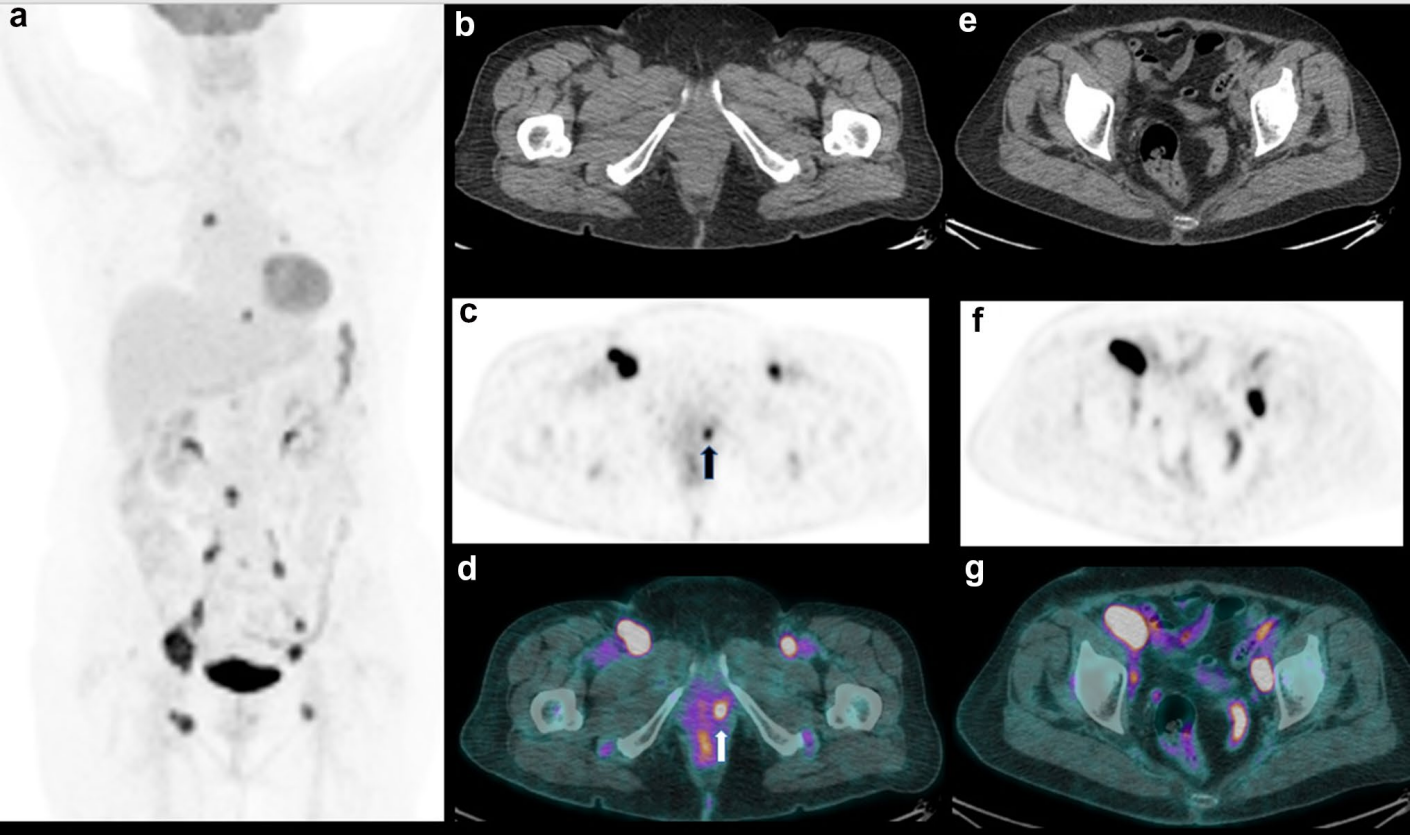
A representative case of positive ^18^F-FDG PET/CT in a 69-year-old woman previously treated with partial vulvectomy. Maximum intensity projection (MIP) (a) showing plural increased FDG uptakes in the pelvis, abdomen and chest. Axial CT (b), PET (c) and PET/CT fused (d) images demonstrating uptake corresponding to left vulvar lesion (black arrow) and some bilateral groin nodes. Axial CT (e), PET (f) and PET/CT fused (g) images revealing bilateral iliac nodes
